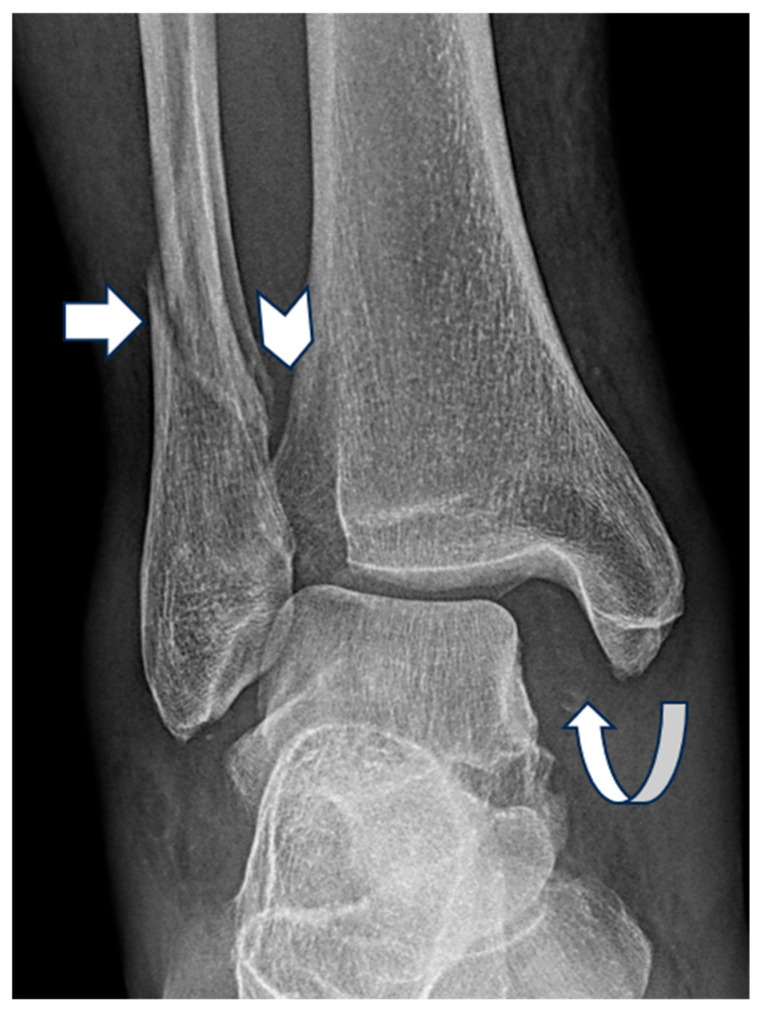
Mortise view radiograph of the right ankle demonstrating a displaced Weber C lateral malleolar fracture (white arrow) with widening of the medial clear space (curved arrow) and lateral clear space (chevron) but normal superior clear space.
